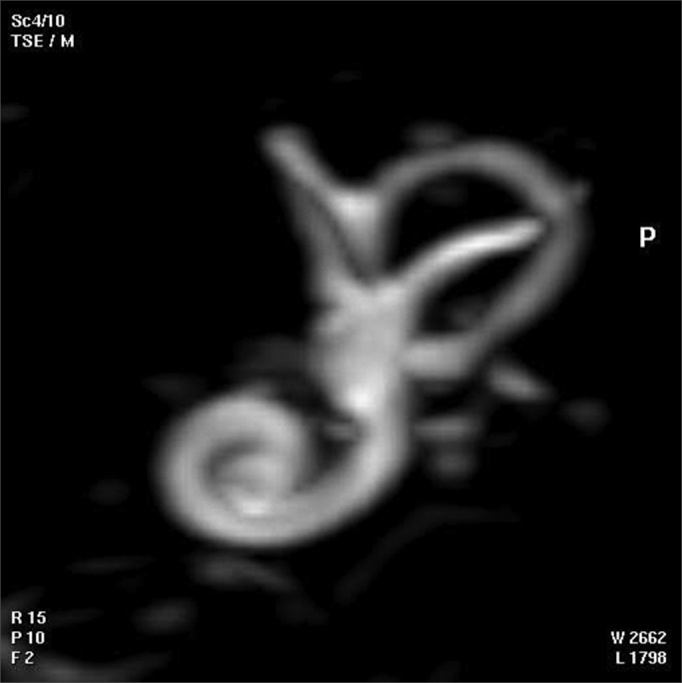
3D magnetic resonance image chosen for providing the best image of the turns of the spiral canal of cochlea in the temporal bone of case 1.
